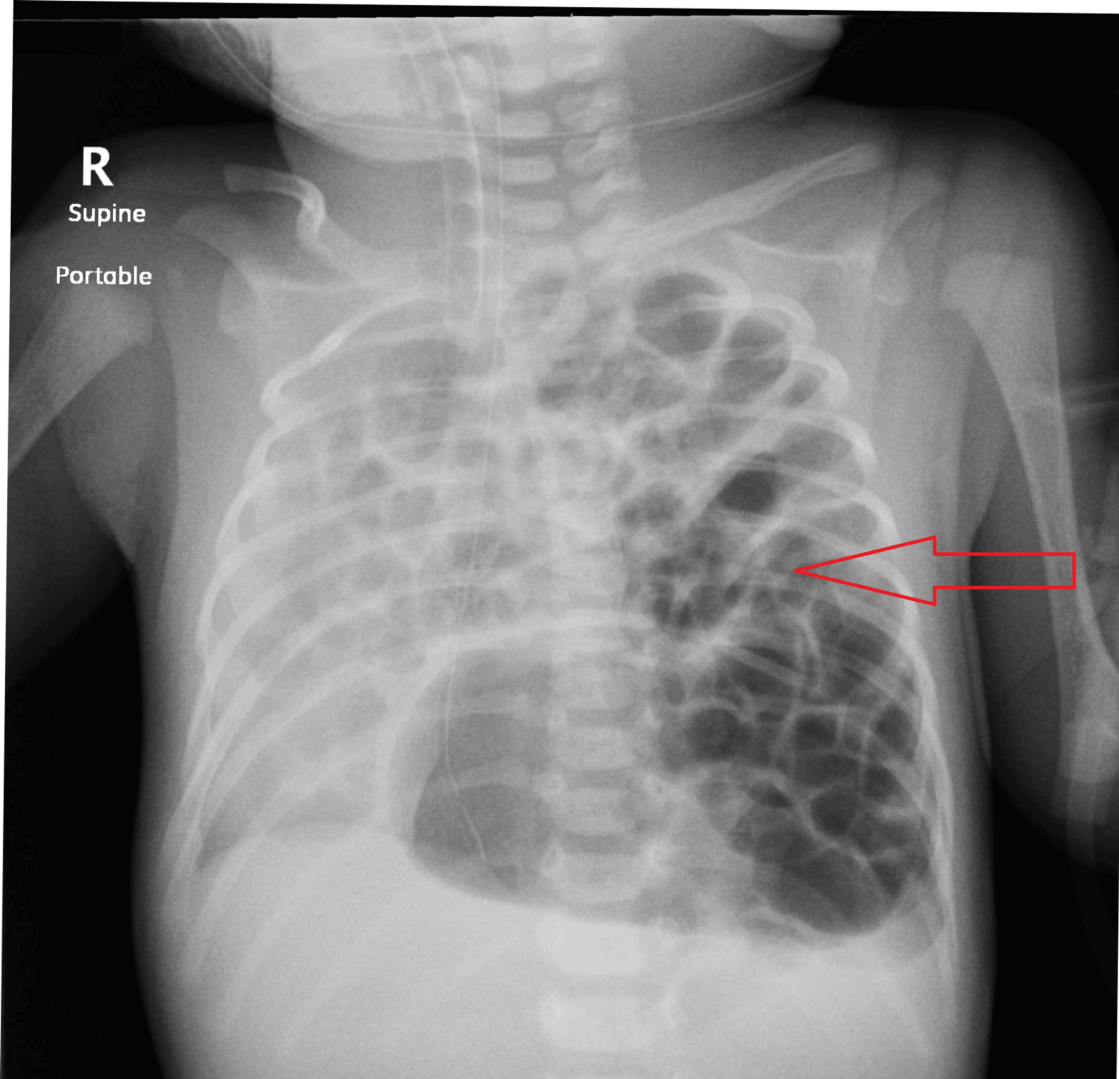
Postnatal chest X-ray taken post-delivery, demonstrating bowel loops herniating into the left hemithorax (arrow), with right mediastinal shift.
